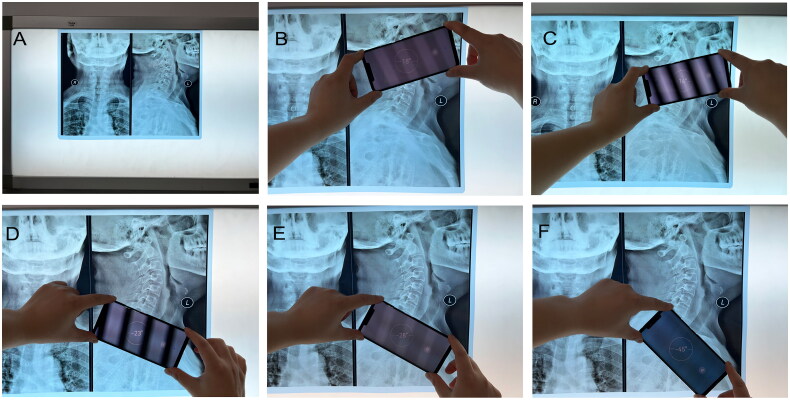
Measurement of the represented angles by smartphone’s intrinsic inclinometer. A. X-rays were placed on the film viewer, which was placed on the wall. B. Confirm the McGregor line, align a certain edge of the smartphone to the McGregor line, and record the angle displayed on the smartphone screen. C. Confirm the lower endplate of C2, align the same edge of the smartphone to the lower endplate of C2, and record the angle displayed on the smartphone screen. D. Confirm the lower endplate of C7, align the same edge of the smartphone to the lower endplate of C7, and record the angle displayed on the smartphone screen. E. Confirm the upper endplate of T1, align the same edge of the smartphone to the upper endplate of T1, and record the angle displayed on the smartphone screen. F. The line connecting the upper end of the sternum and the center of the T1 upper endplate aligned the same edge of the smartphone to this line and recorded the angle shown on the smartphone screen.
